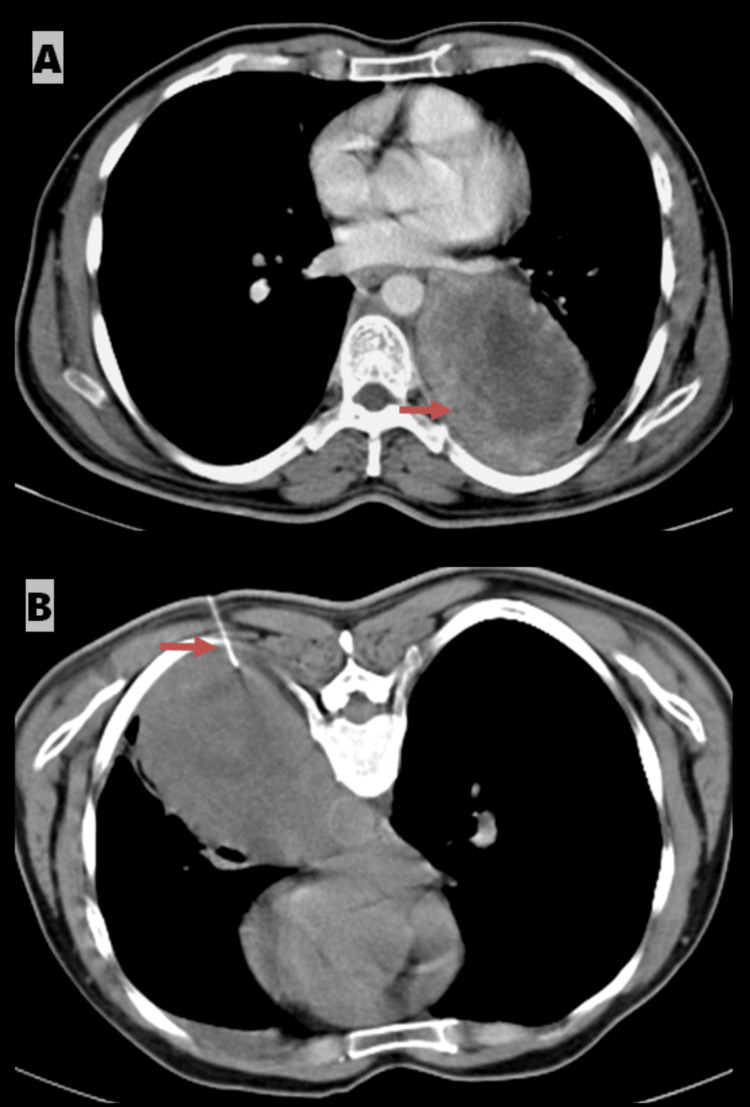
A) Axial contrast-enhanced CT in the mediastinal window showing a heterogeneous lesion in the left lower lobe (indicated by an arrow). B) Prone axial CT image demonstrating the biopsy needle within the mass in the left lower lobe (indicated by an arrow).
